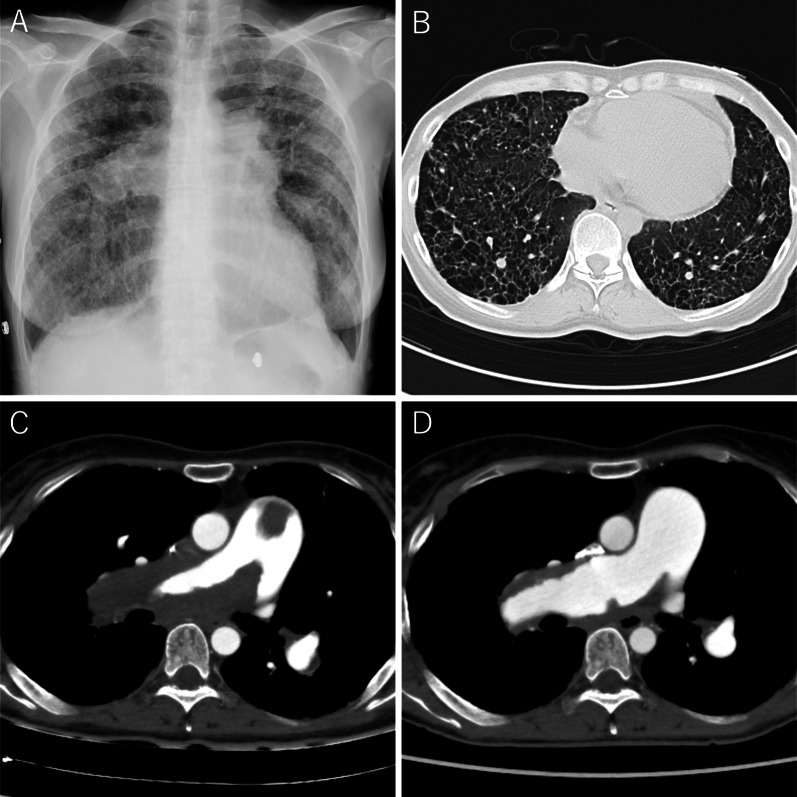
Radiological images. A Bilateral enlarged pulmonary artery shadows are seen in the chest radiograph. B The computed tomography shows numerous thin-walled pulmonary cysts. C Massive thrombi in dilated pulmonary artery extending from the pulmonary trunk to both main pulmonary arteries are revealed at initial enhanced computed tomography. D Follow-up computed tomography after 3 months of anticoagulation therapy reveals markedly shrinking thrombi limited in the main pulmonary artery wall
